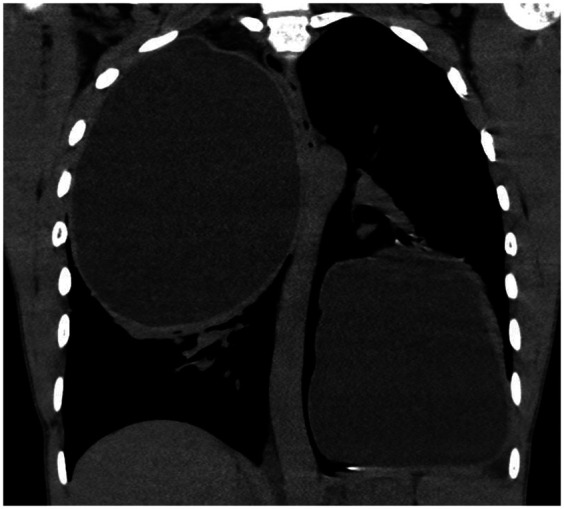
Computed tomography (CT) scan of the chest (coronal section) showing bilateral large cysts with homogenous fluid density and peripheral rim enhancement, suggesting bilateral giant hydatid cysts.
